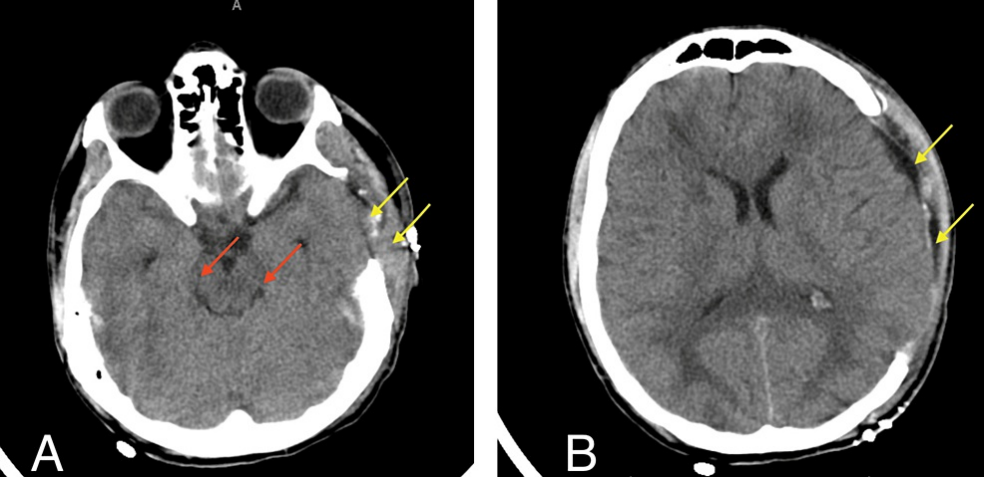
Axial Head CT after DHC demonstrating adequate decompression of cisterns (red arrows), and craniectomy (yellow arrows) DHC: decompressive hemicraniectomy
